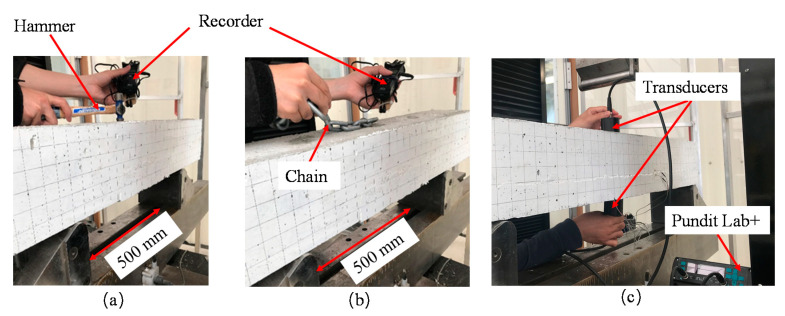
Experimental setup of (a) Hammer percussion test (b) Modified chain drag test (c) Ultrasonic pulse velocity test.
